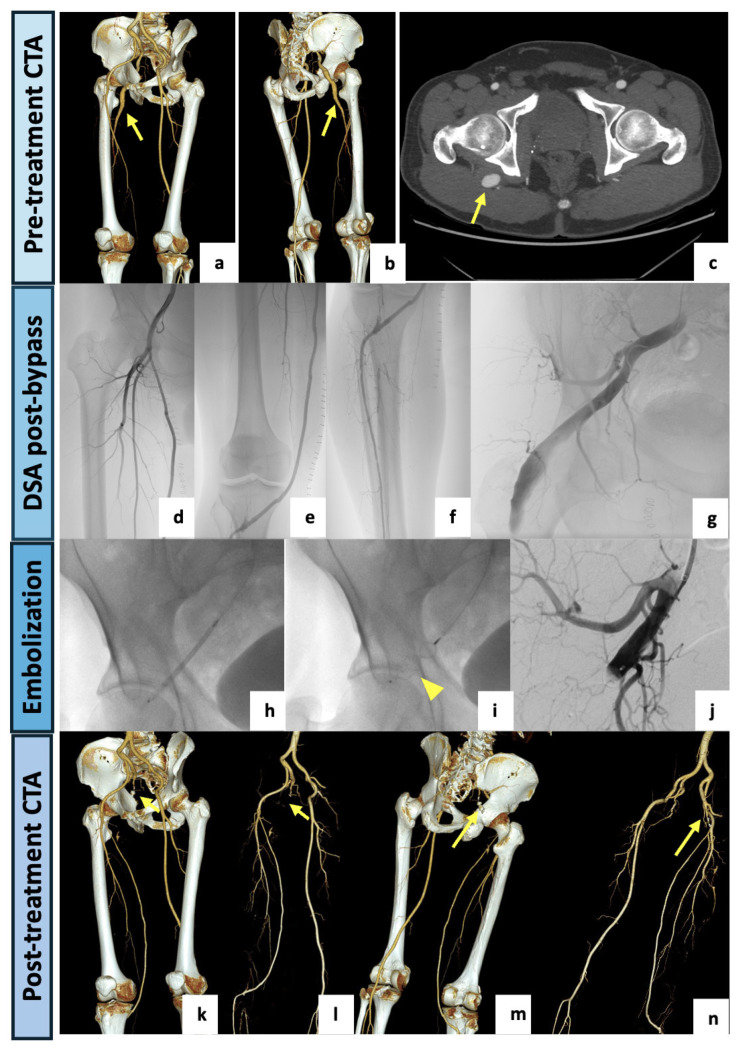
(a,b) VR reconstruction of the preoperative CTA showing a right complete PSA originating from the internal iliac artery (arrow). The right external iliac artery appears hypoplasic and the SFA appears incomplete and diminished in caliber (type 2a). (c) Axial view showing an aneurysmal dilatation (23 mm) of the PSA with luminal thrombus (arrow). A hybrid approach was preferred, with the first step consisting of a left femoro-tibial bypass with the anterior tibial as the target artery. (d–f) Selective angiographic images preliminary to the endovascular step showing a patent femoro-tibial bypass. (g–j) After a contralateral 6F-sheat femoral access, the aneurysmal PSA was selectively catheterized and completely embolized using a 12 mm Amplatzer Vascular Plug II (arrowhead) deployed immediately distally to the internal pudendal artery origin. (k–n) VR reconstructions of the postoperative CTA (3-month) demonstrating the patent femoro-tibial bypass with complete exclusion of the aneurysmal PSA (arrows).
